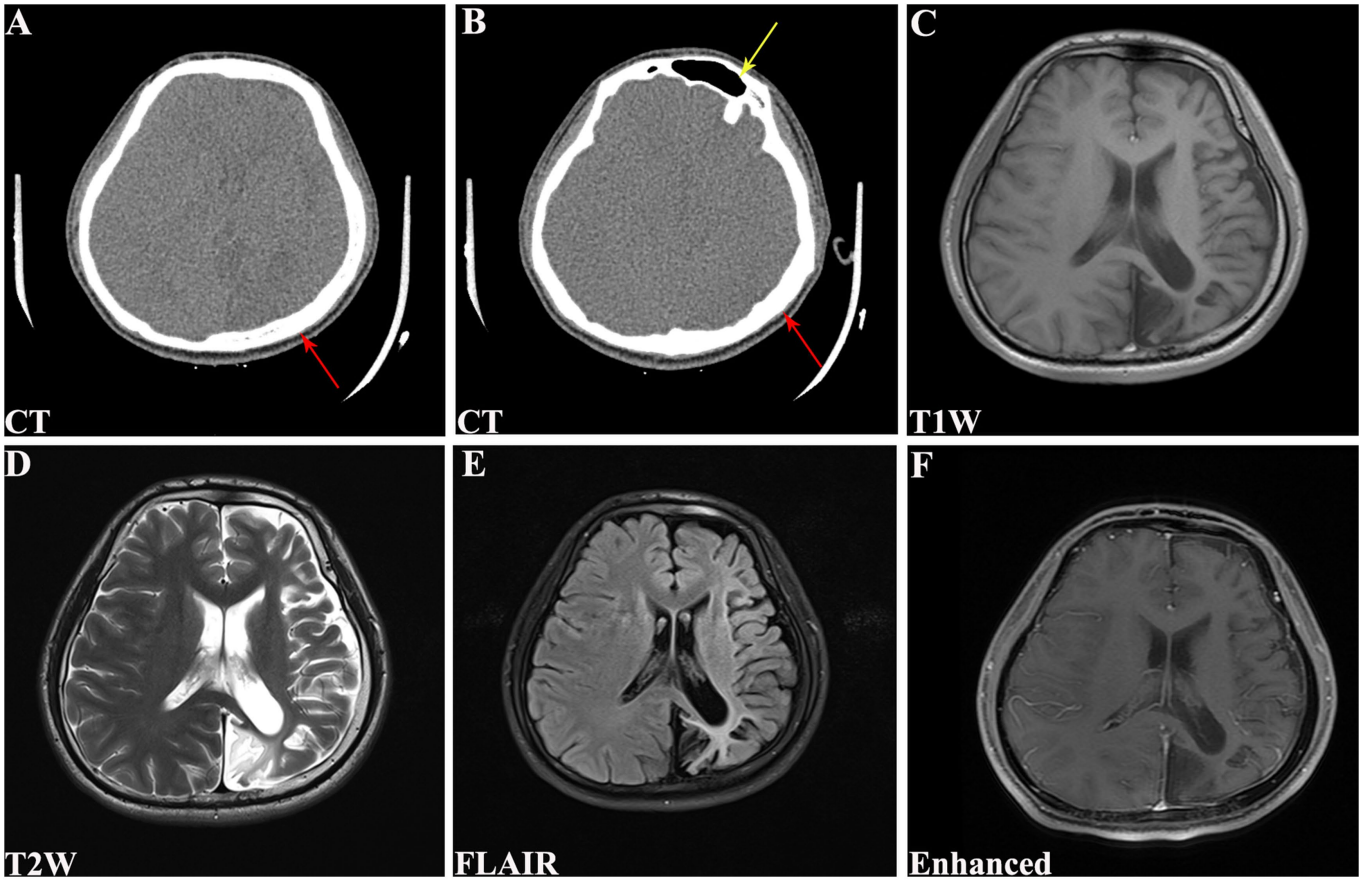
Cranial CT and MRI scans of the patient. (A) CT revealing left hemisphere atrophy with Compensated cranial thickening (red arrow). (B) CT demonstrating hyperpneumatization of the left frontal sinus (yellow arrow) with compensated cranial thickening (red arrow). (C) T1W, (D) T2W, (E) FLAIR, and (F) enhanced MRI showing left cerebral hemiatrophy along with enlargement of ipsilateral lateral ventricle. CT, computed tomography; MRI, magnetic resonance imaging; T1W, T1-weighted; T2W, T2-weighted; FLAIR, fluid attenuated inversion recovery.
